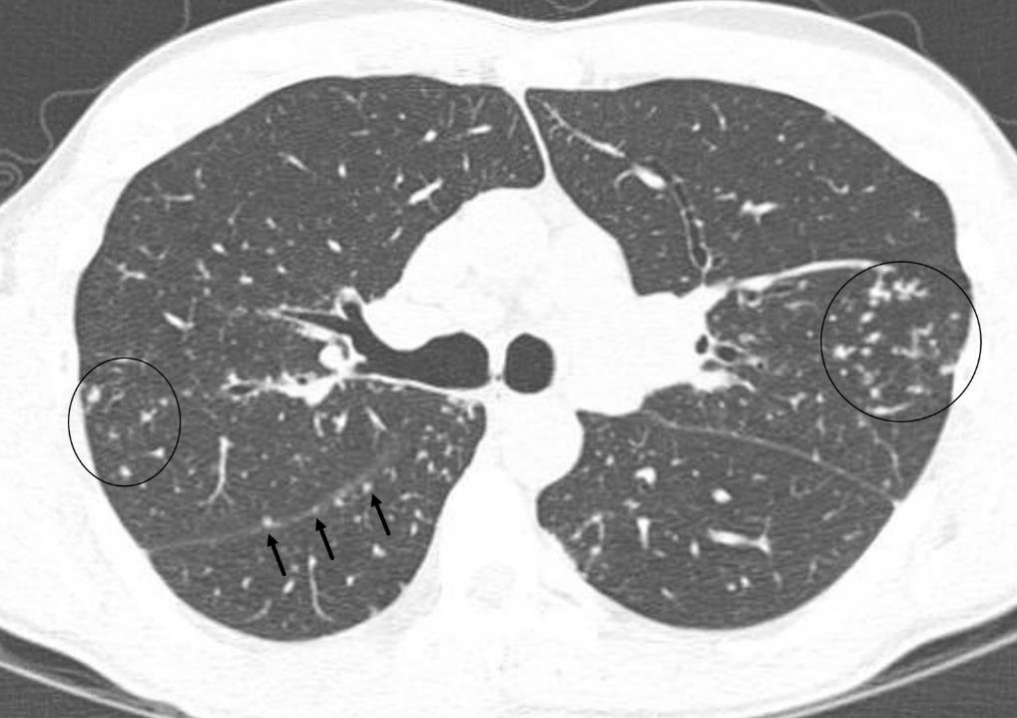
An axial CT image (lung window) of a 41 years old female with sarcoidosis shows cluster of nodular and branching opacities (circled) in the lateral aspect of upper lobes of both the lungs. A few of these opacities abut the pleural surface of lung. Note presence of small nodules along the oblique fissures particularly pronounced in the right lung (arrows).
